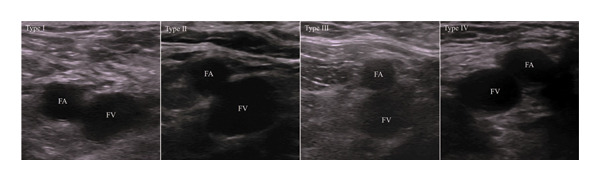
Topographical classifications of femoral arteriovenous spatial relationships. Note: Type I: parallel venoarterial orientation, no overlap. Type II: medial venous position, ≤ 50% luminal overlap. Type III: medial venous position, > 50% overlap. Type IV: lateral venous course. All ultrasound images presented in the figure were derived exclusively from study participants, with documented informed consent obtained. Abbreviations: FA: femoral artery; FV: femoral vein.
